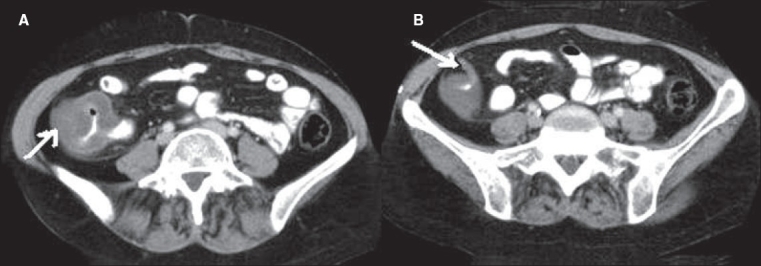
Colitis. Contrast-enhanced CT scan shows circumferential wall thickening of the ascending colon and cecum with surrounding fat stranding (arrow in Figure A). The terminal ileum and appendix (arrow in Figure B) are normal
